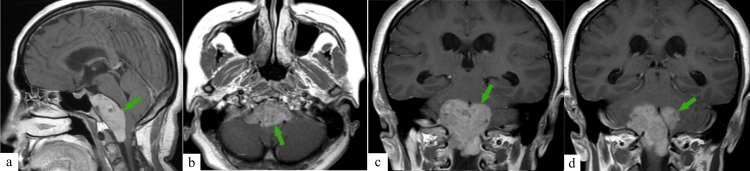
MRI of the brain before endoscopic transnasal transclival tumor removal. T1-weighted images with contrast enhancement (the tumor is indicated by the green arrows): (a) sagittal plane; (b) axial plane; (c and d) coronal plane. Tumor dimensions 61 mm x 50 mm x 32 mm (length x width coronal x width sagittal). MRI, magnetic resonance imaging
